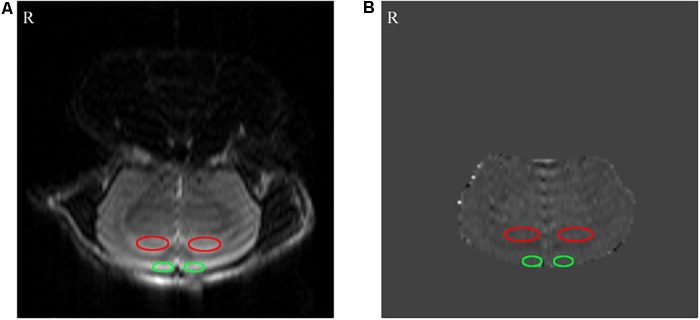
The results of the arterial spin labeling (ASL) MRI. (A) Perfusion FAIR-RARE images. (B) CBF images. The bilateral hippocampus and prefrontal lobe are shown by red and green ellipses, respectively.
